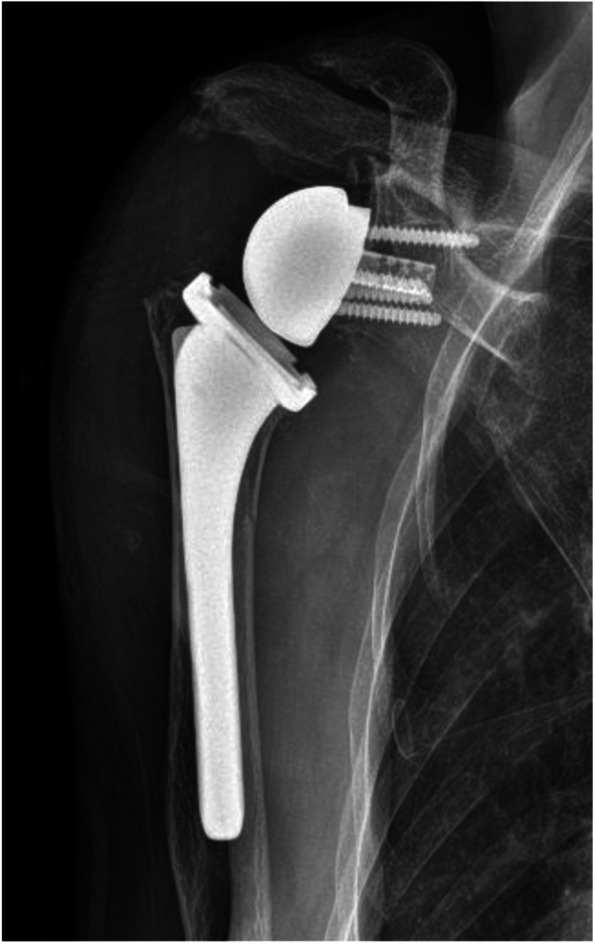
Postoperative anteroposterior radiograph of the right shoulder demonstrating a reverse total shoulder arthroplasty
